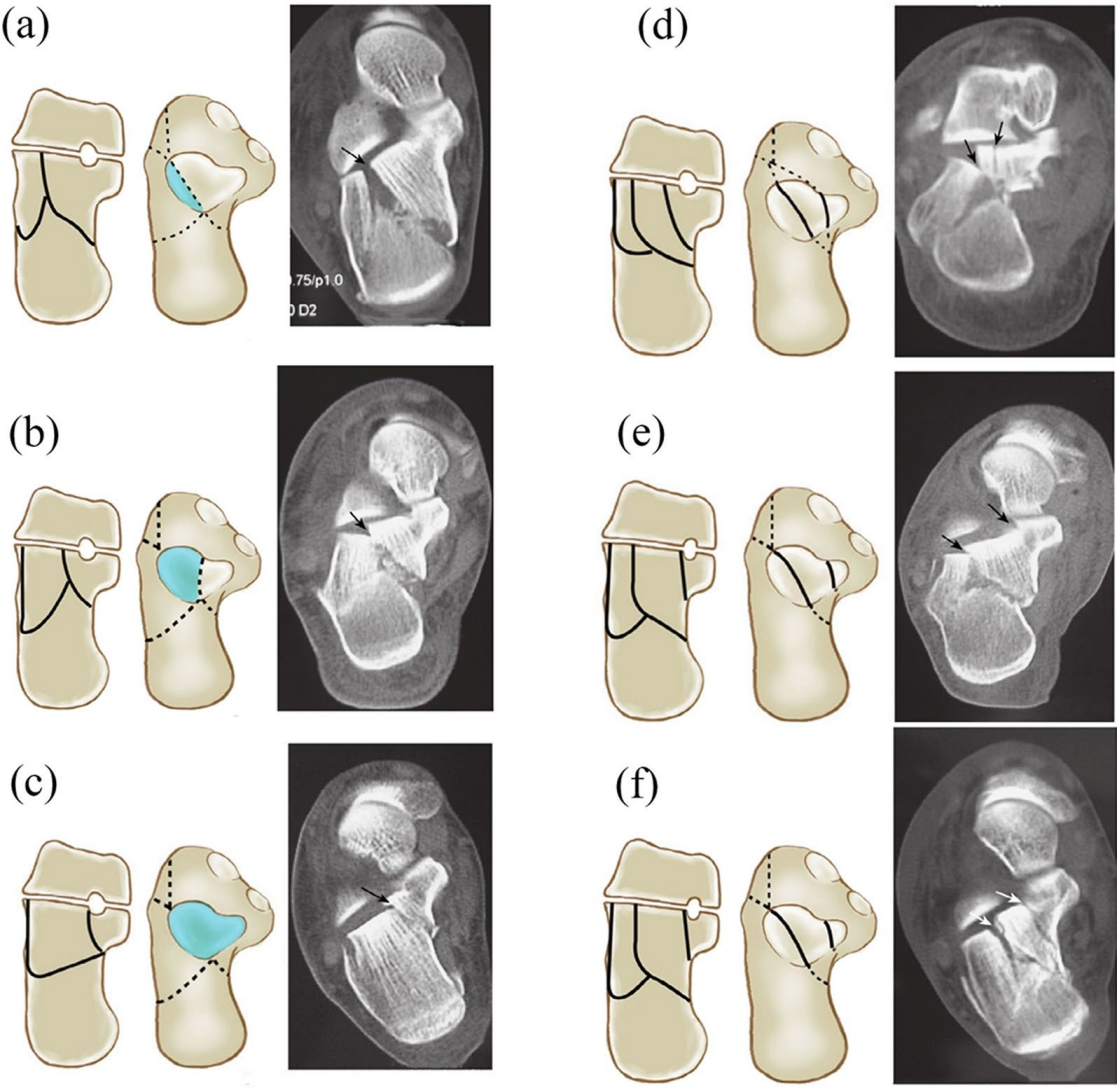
The CT images of Sanders type II and type III calcaneal fractures a IIa: fracture line lateral to the fracture line; b IIb: fracture line medial; c IIc: fracture line adjacent to the sustentaculum tali; and d, e and f three-part fracture of the posterior talar articular surface of type III: IIIab, IIIac, IIIbc
